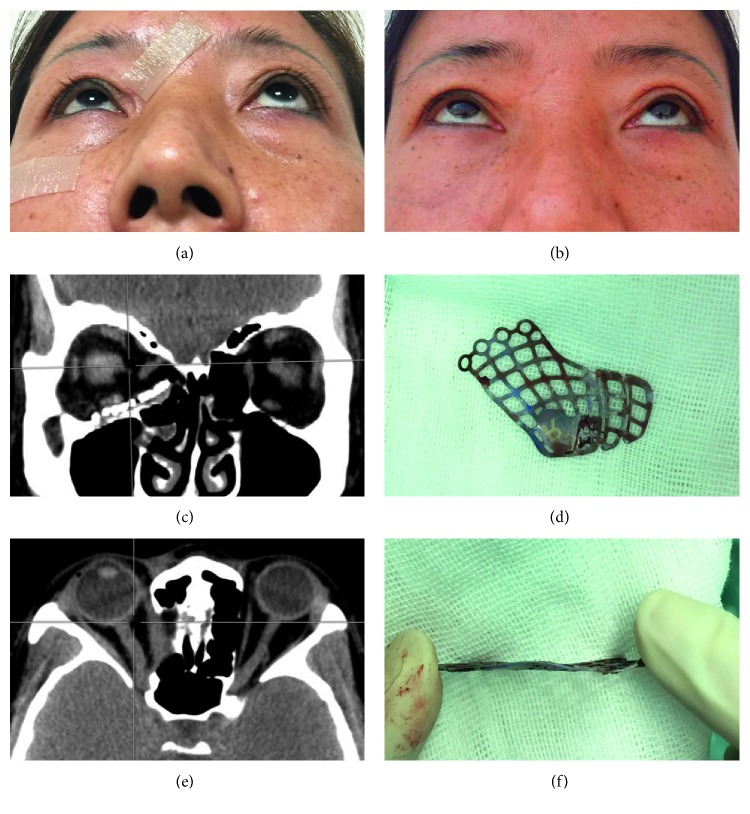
Case of a malpositioned titanium mesh implant. (a) Enophthalmos 1 month after previous orbital fracture repair. (b) The enophthalmos was fully corrected 6 months after secondary implantation of RapidSorb. (c and e) CT images showing that the implant did not fully cover the medial wall defect, causing herniation of orbital contents into the ethmoid sinus. (d and f) The explanted titanium mesh was flat and did not conform to the orbital anatomical structure.
